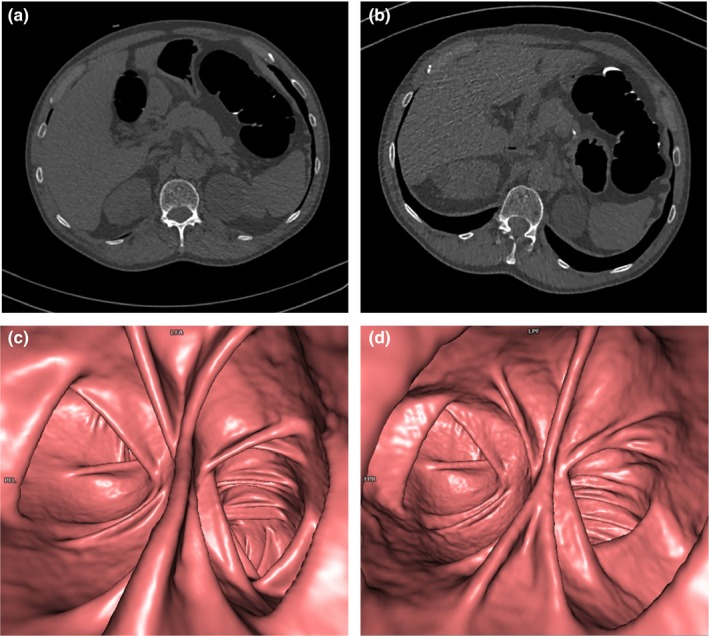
Axial two‐dimensional (2D) computed tomography images (a,b) and three‐dimensional (3D) endoluminal reconstructions (c,d) of the splenic flexure in a 71‐yr‐old, class B, man after incomplete colonoscopy. Both 2D low‐dose (a) and ultra‐low dose (ULD) (b) images were rated to have comparable overall image quality, despite a slight increase of the perceived noise and aortic contour blurriness of the ULD protocol. Similarly, no significant differences were found between overall 3D image quality scores of ULD and LD acquisitions, despite a slight increase of the mural surface irregularity of the ULD protocol (d).
